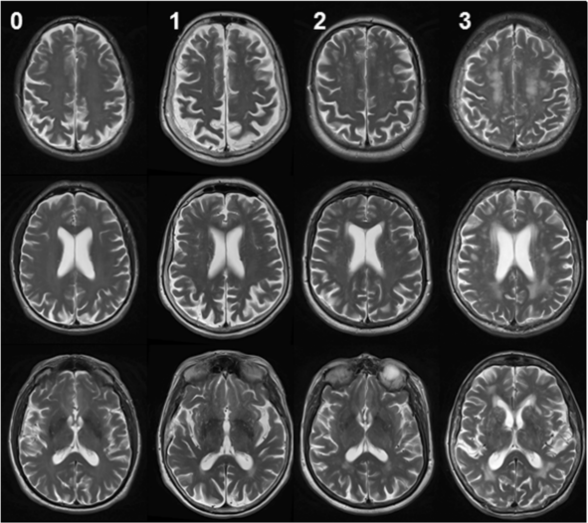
Examples of typical magnetic resonance imaging of white matter disease. *White matter lesions*. Usually attributed to chronic small vessel ischaemia, rating using the Fazekas scale. No lesions or single punctate lesion (grade 0), multiple punctate lesions (grade 1), beginning confluency of lesions (bridging, grade 2), large confluent lesions (grade 3)
